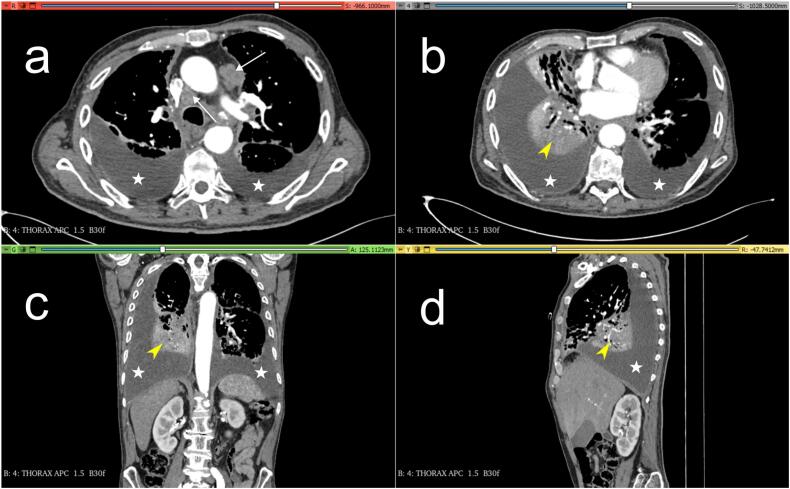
CT scan images. (a) and (b) axial views, (c) coronal view and (d) sagittal view, showing consolidation in the independent portion of the right lower lobe (Yellow arrow heads), bilateral pleural effusion (white stars), and lymphadenopathies (White arrows in (a)). (For interpretation of the references to colour in this figure legend, the reader is referred to the web version of this article.)
